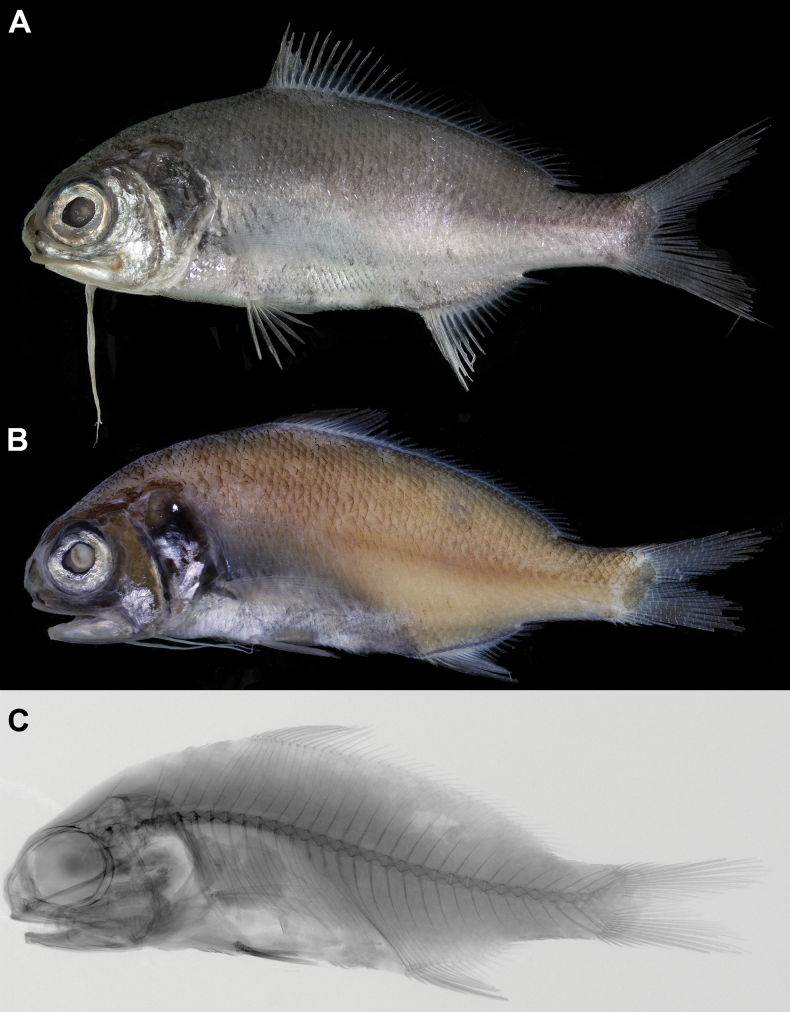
Polymixiamelanostoma* sp. nov., holotype, NMMB-P39587, 110.2 mm SLA fresh B preserved C x-radiograph.
